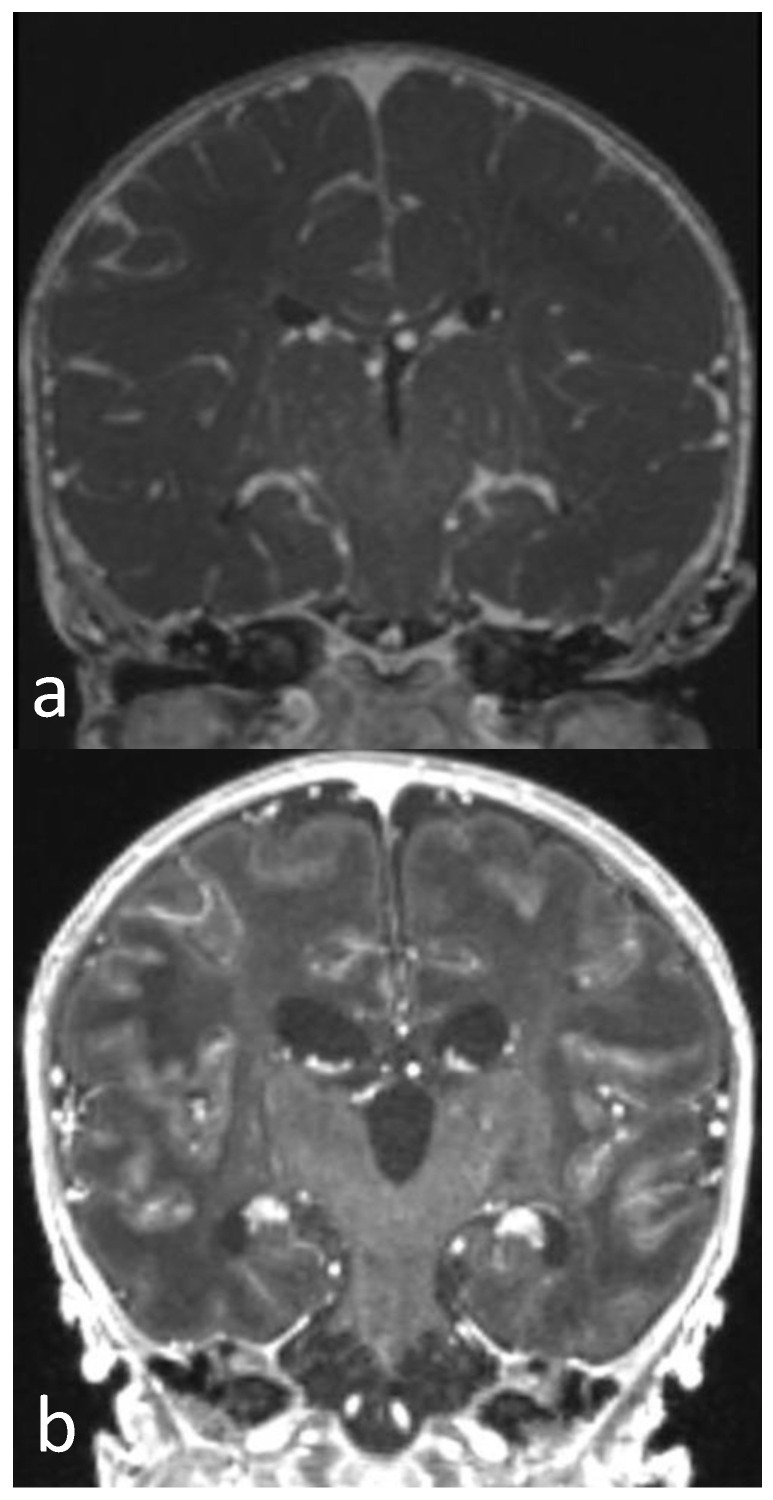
Post-contrast T1WI sequence performed 3 (a) and 12 days after birth (b). Diffuse and linear leptomeningeal enhancement in first MRI (a) persists in follow-up MRI (b). Diffuse brain swelling (a) evident in first MRI is markedly reduced in second MRI (b), in which a reduction in brain oedema and dilatation of ventricular system and subarachnoid spaces are shown.
